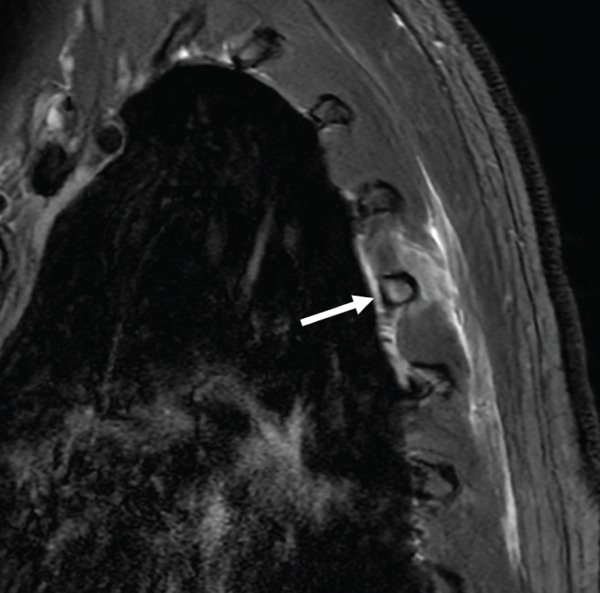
Sagittal T2‐weighted fat‐suppressed MR image of the left thoracic wall. There is bone marrow edema of the fifth left rib (arrow), with surrounding soft tissue edema.
